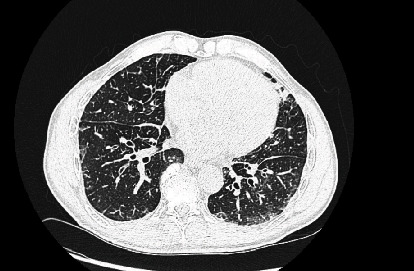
CT chest without contrast showing interlobular septal thickening, bronchiectasis, and basilar predominant lung opacities.
